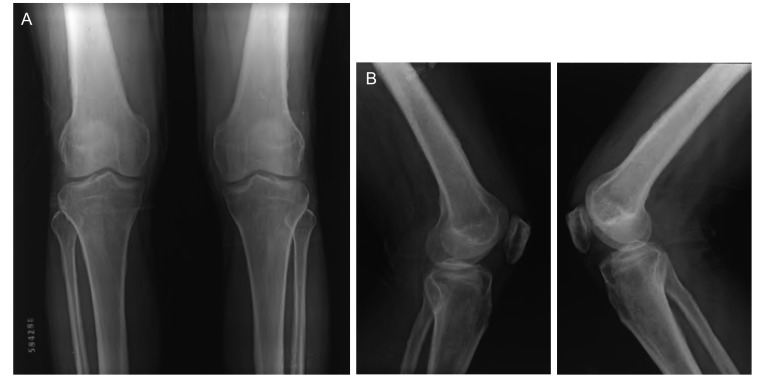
双膝关节X线检查。双侧股骨远端、胫骨上端及腓骨近端可见对称性骨膜增生。A：正位；B：侧位。 X-ray of both knees. The distal part of bilateral femur and the proximal part of tibiaand fibula show symmetry periosteal proliferation. A: normotopia; B: lateral.
